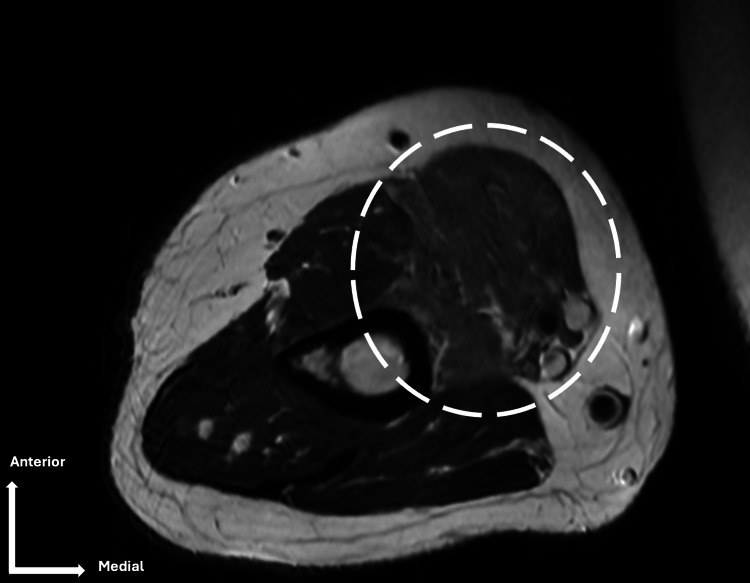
Axial MRI (T2) Hypersignal in the biceps brachii muscle (white circle). MRI: magnetic resonance imaging
